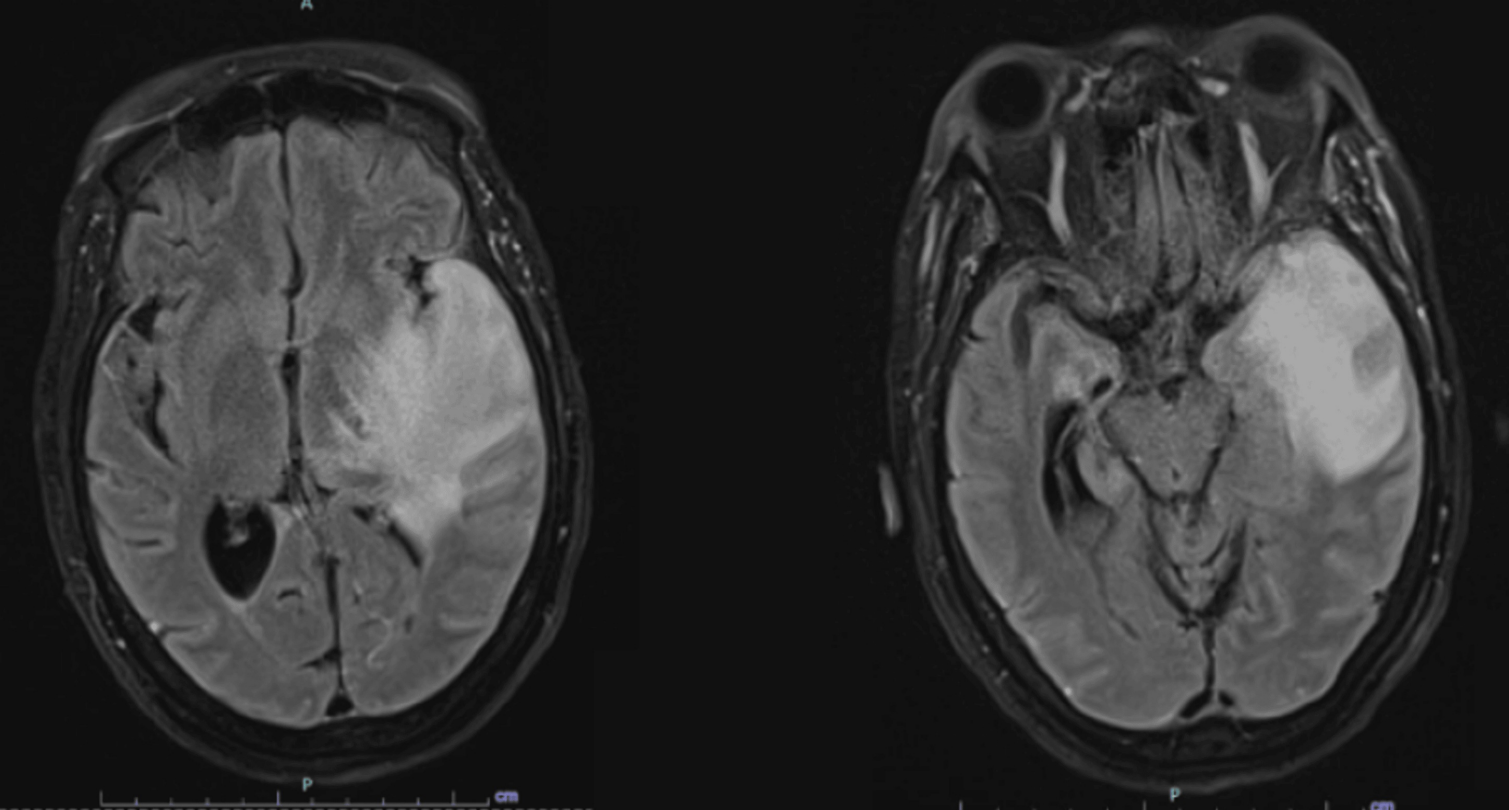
Initial MRI brain Findings of hyperintense areas on T2-weighted imaging, fluid-attenuated inversion recovery (FLAIR) accompanied by gadolinium enhancement on T1-weighted imaging in regions including temporal lobes, cingulate gyrus, orbitofrontal cortex, and insular cortex.
